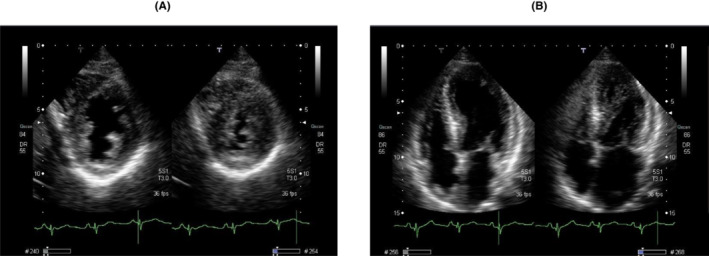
(A, B) Echocardiography showed a markedly increased left ventricular wall thickness with reduced contractions in posterior and lateral regions.
